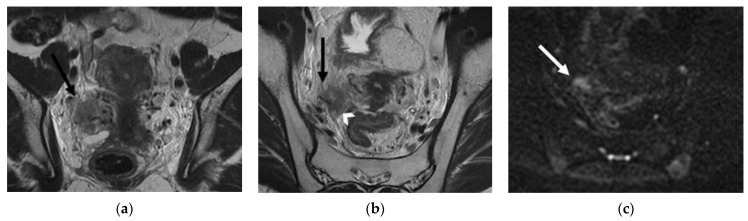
A 32-year-old woman who underwent previous chemoradiation therapy for cervical cancer. (a) Coronal and (b) axial FSE T2-weighted images show a soft-tissue mass in the right parametrium (black arrows). Note the contact with the right ureter embedded by the lesion (arrowhead). (c) Diffusion-weighted image shows focus of hyperintense signal into the lesion (white arrow). The finding was confirmed to be recurrence at surgery.
